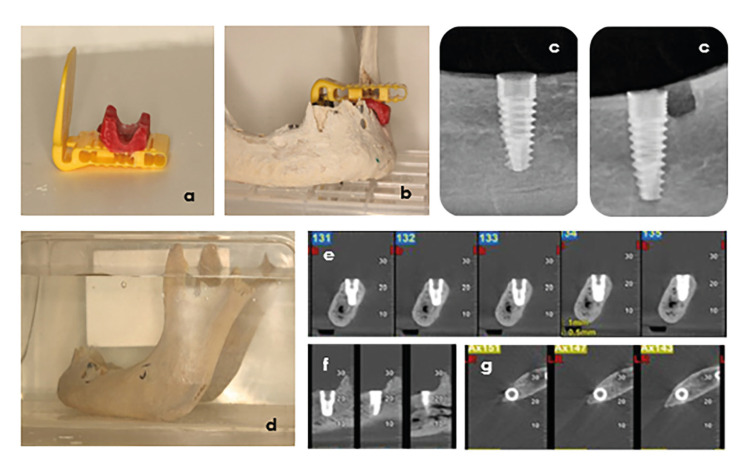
Acrylic matrix used (a,b) to maintain repeatable and stable conditions when taking periapical radiographs (c). The mandible was placed in a plastic container filled with water (d) prior to CBCT examination to imitate the X-ray attenuation produced by soft tissues. Example of CBCT images obtained in cross-sectional (e), sagittal (f), and axial (g) planes.
